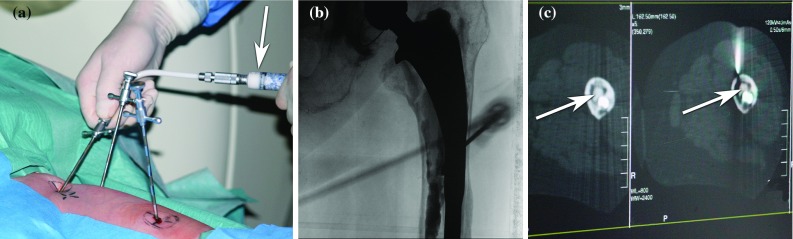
Percutaneous cement injection can be monitored by looking at a the syringe’s plunger, b fluoroscopic projections or c intraoperative cross-sectional CT slices
